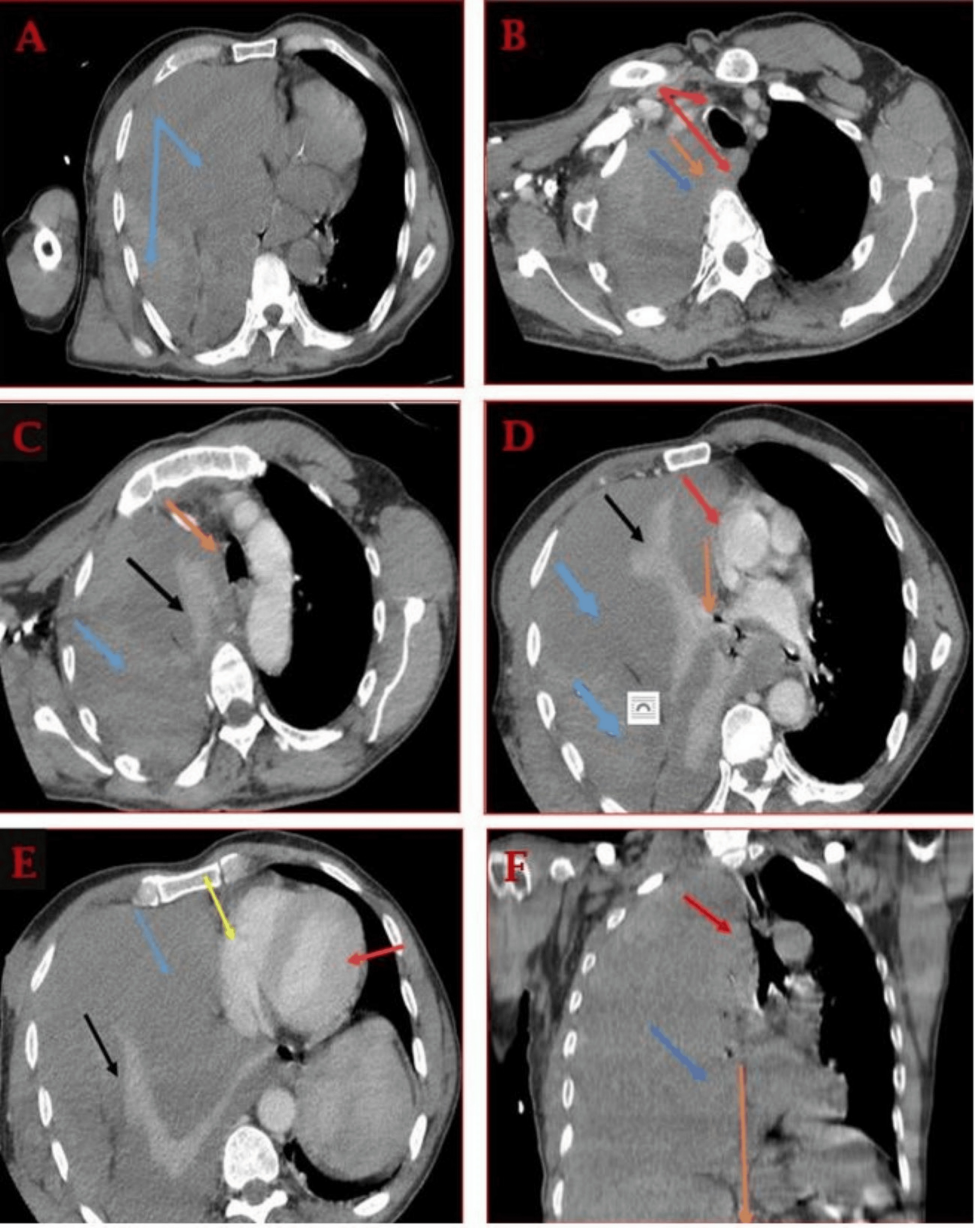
Chest CT scan before and after contrast enhancement in axial and coronal views shows a large, right heterogeneous pleural effusion with spontaneously hyperdense areas (blood) (A-E) and darker areas (fluid), creating a fluid-blood level suggestive of a massive hemothorax (blue arrows) (A-F). Possible mediastinal lymph nodes are noted in the upper mediastinum between the opacified supra-aortic trunks (B, D) and behind the trachea (B) (red arrows). Pulmonary collapse is also observed at the right pulmonary hilum (C-E) (black arrow). There is a significant mass effect exerted by the right hemothorax on the mediastinal structures (B, C, D) and heart (yellow arrow) (E), which are displaced toward the contralateral side with no visible pericardial effusion (E) (red arrow).
